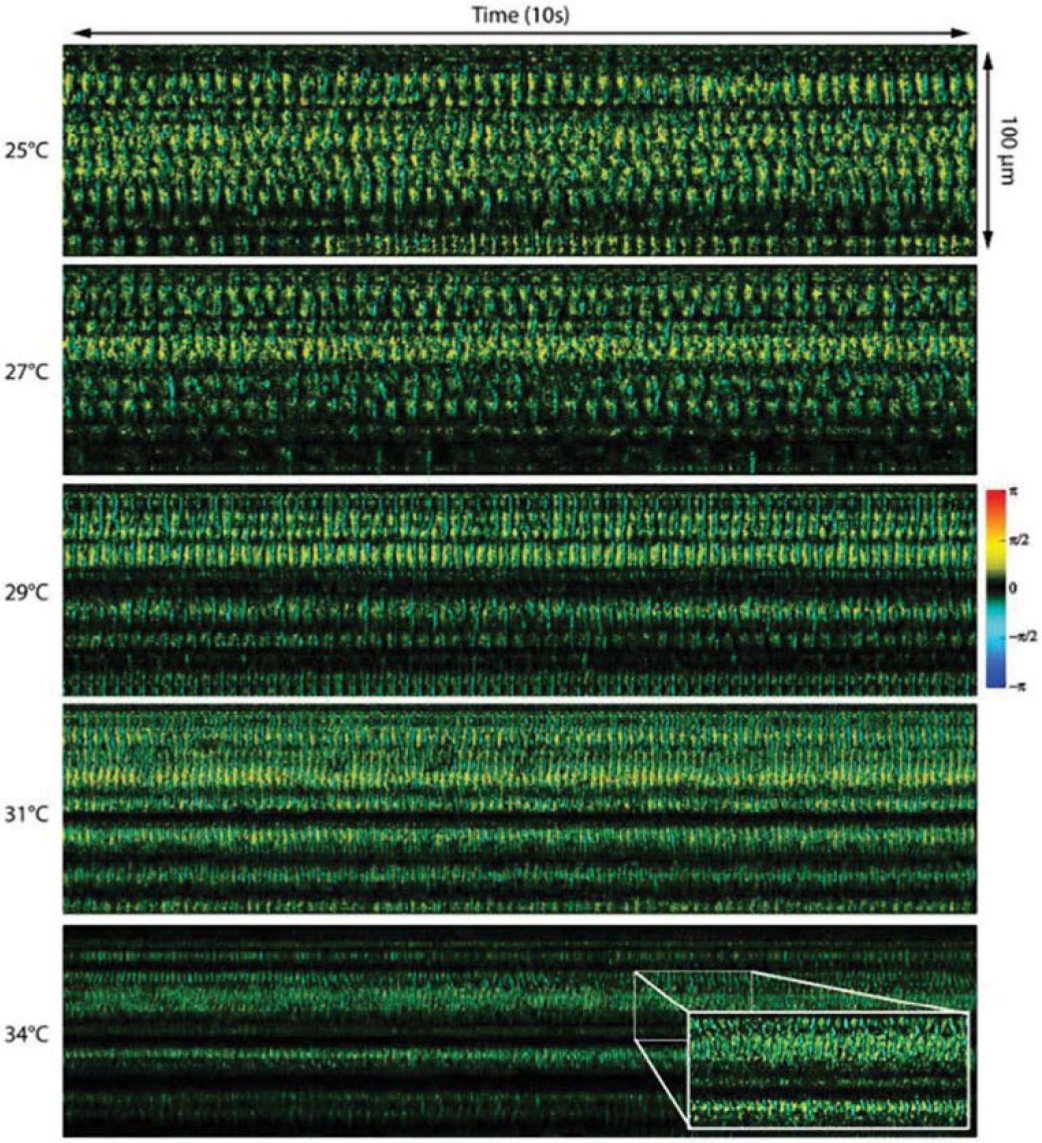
Visualization of cilia beating frequency at different temperatures using Doppler OCT. Cilia beats faster as the temperature increases. Reprinted with permission from ref. [51].
